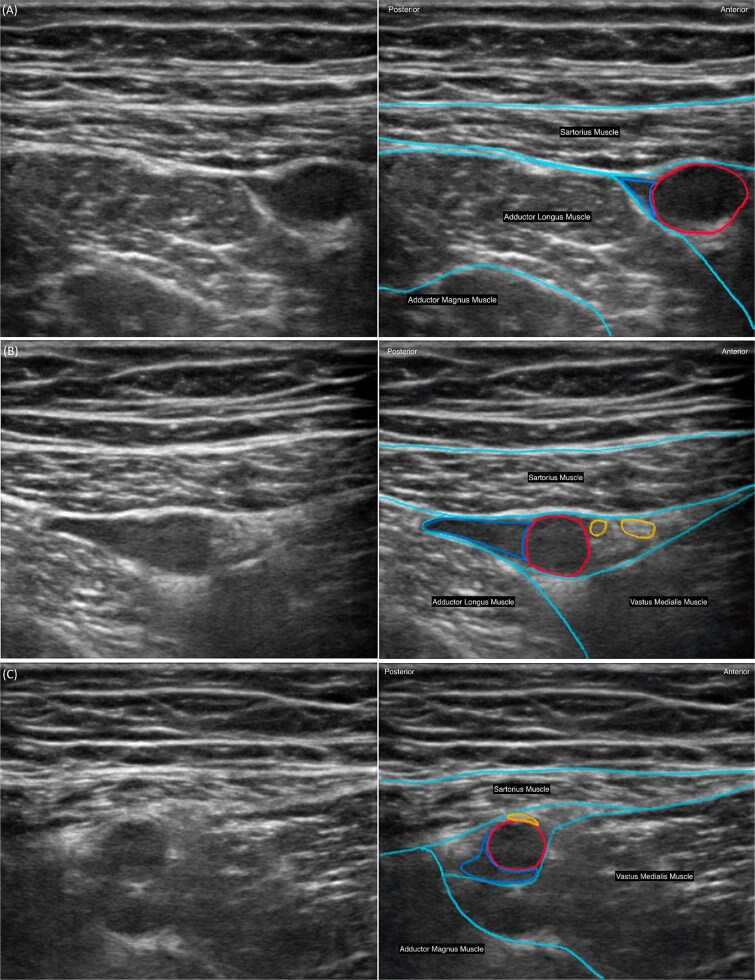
Ultrasound image of the adductor canal blocks. (A) Distal femoral triangle, the apex of the femoral triangle recognized by the point at which the medial border of the sartorius muscle crosses the medial border of the adductor longus muscle. (B) Proximal adductor canal, immediately distal to the distal femoral triangle, within the subsartorial aponeurotic compartment of the adductor canal. (C) Distal adductor canal, just proximal to the adductor hiatus, where the femoral artery exits the canal and becomes the popliteal artery. The femoral artery (red) and femoral vein (blue) are highlighted, with muscle borders outlined in cyan. Relevant muscles and bony structures are labeled.
